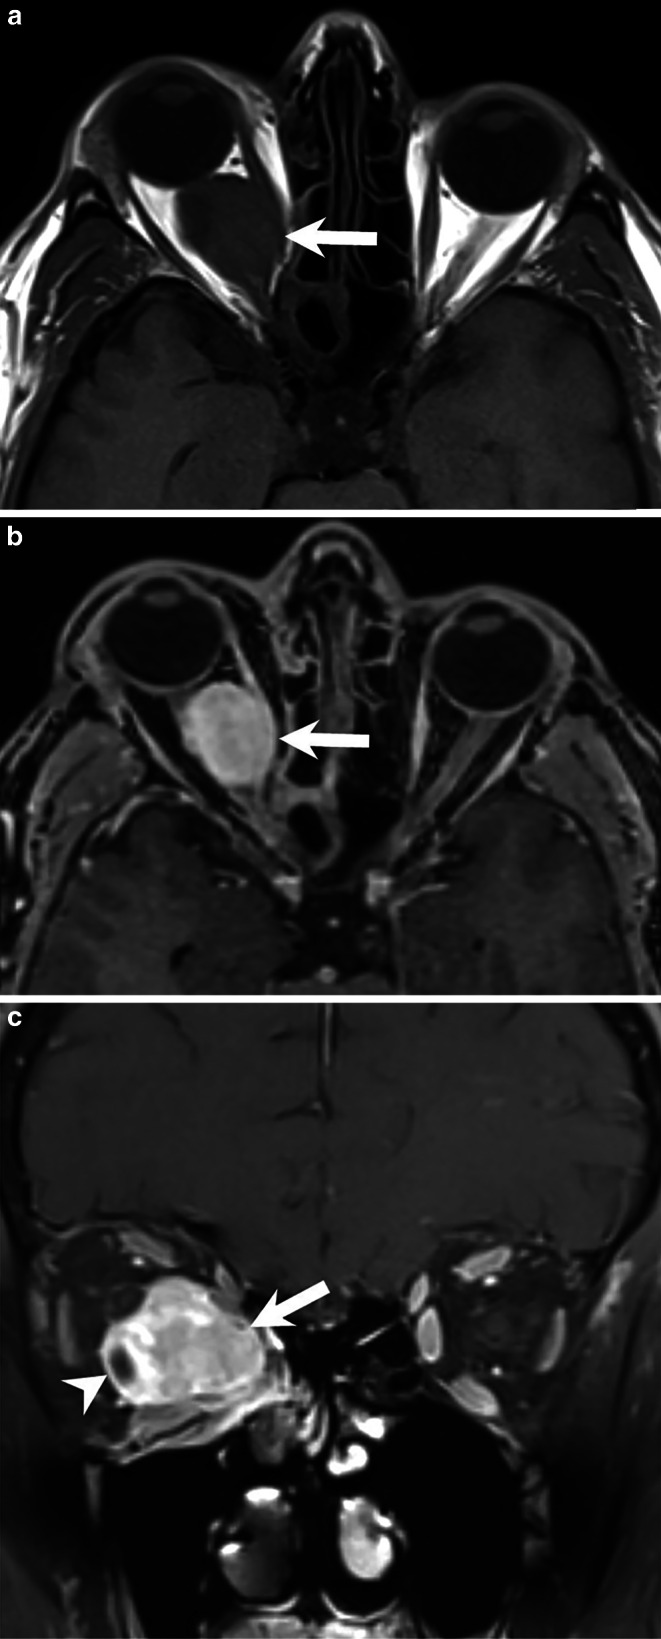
On T1-weighted axial images (a) without contrast the lesion (*arrow*) appeared isointense. On axial (b) and coronal (c) T1-weighted images MRI after administration of Gd the lesion showed homogeneous enhancement of contrast (*arrows*). The cystic portion of the tumor displayed a contrast-enhancing capsule (c, *arrowhead*)
